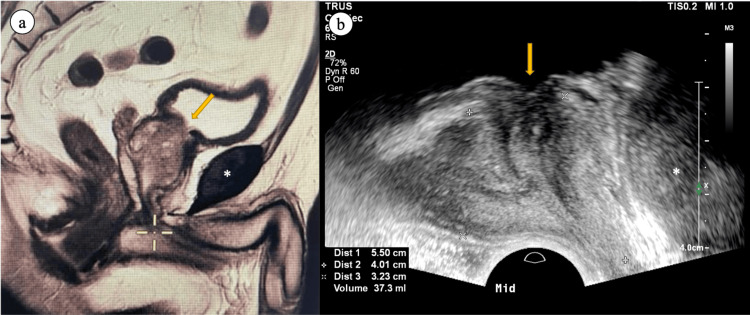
MRI/TRUS fusion using internal plane matching (a) midsagittal image of T2-weighted sagittal MRI sequence with orientation matched; (b) the same midsagittal plane under real-time ultrasound. The bladder neck (arrow) and the pubic symphysis (asterisk) were used as consistent landmarks to facilitate plane matching. Plane registration with fine adjustment was then performed. Abbreviations: MRI=magnetic resonance imaging; TRUS=transrectal ultrasound
